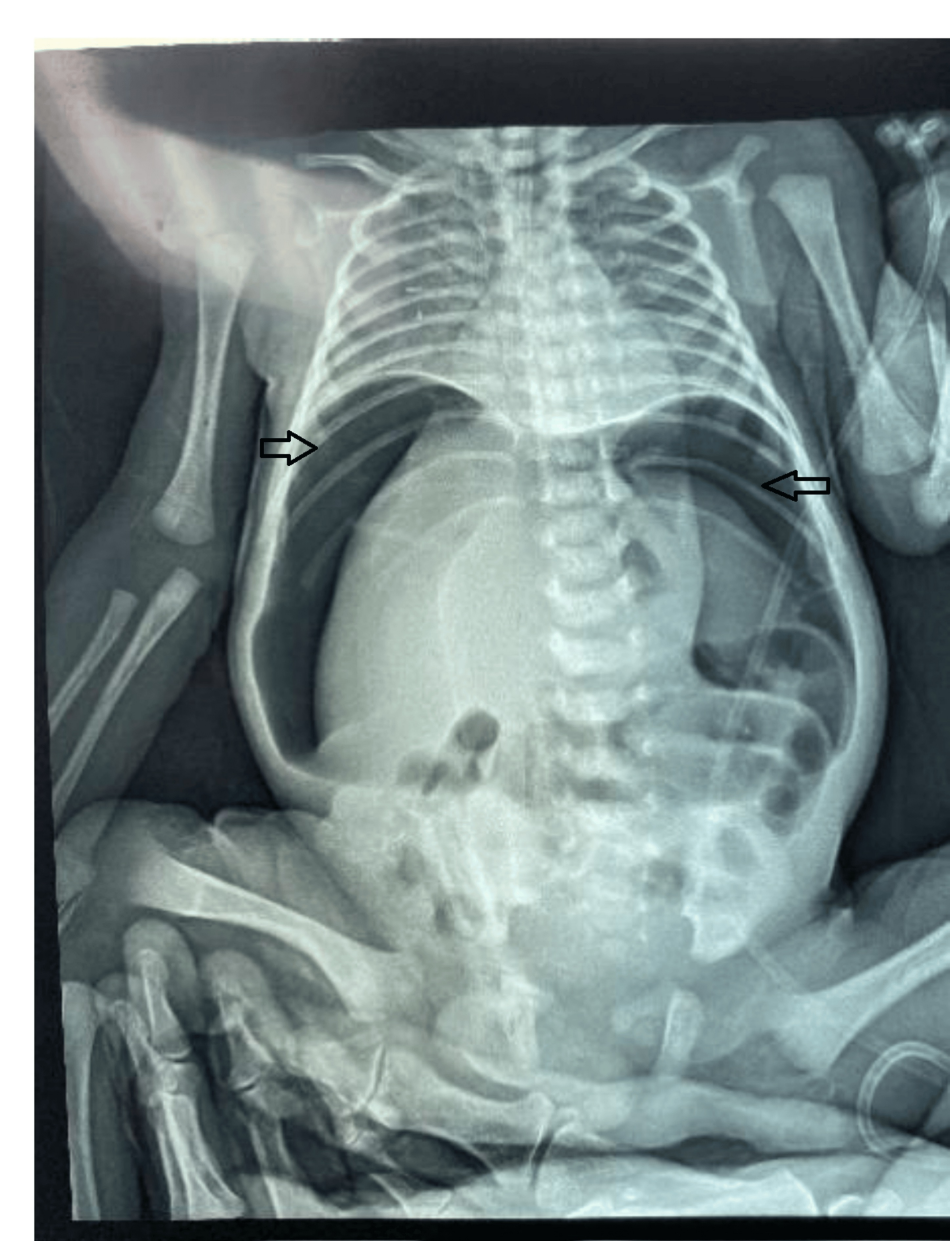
Plain abdominal X-ray demonstrating massive pneumoperitoneum. Note the presence of free air under the diaphragm (indicated by arrows) outlining the abdominal viscera, which is highly suggestive of gastrointestinal perforation
